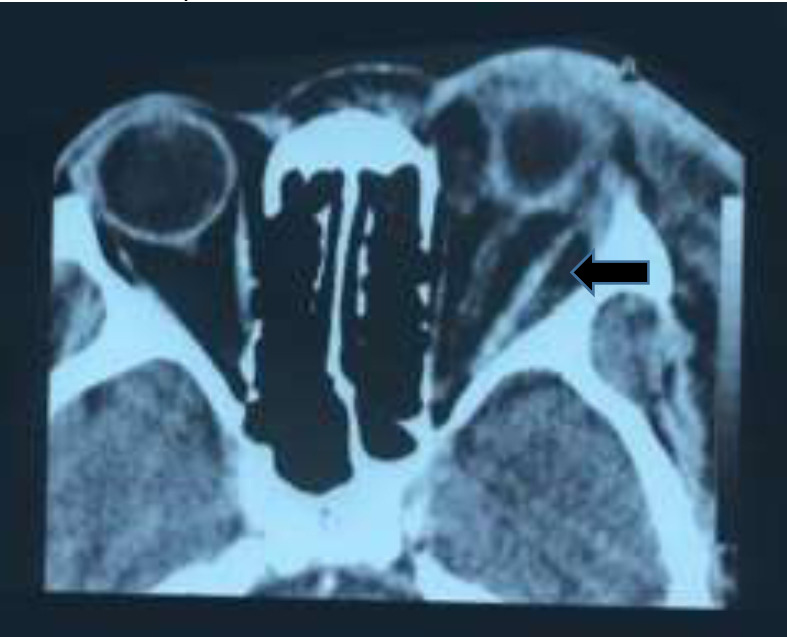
Axial view of CT scan showing orbital sub-tissue swelling and sub-periosteal abscess on the upper lateral orbital wall (black arrow).
